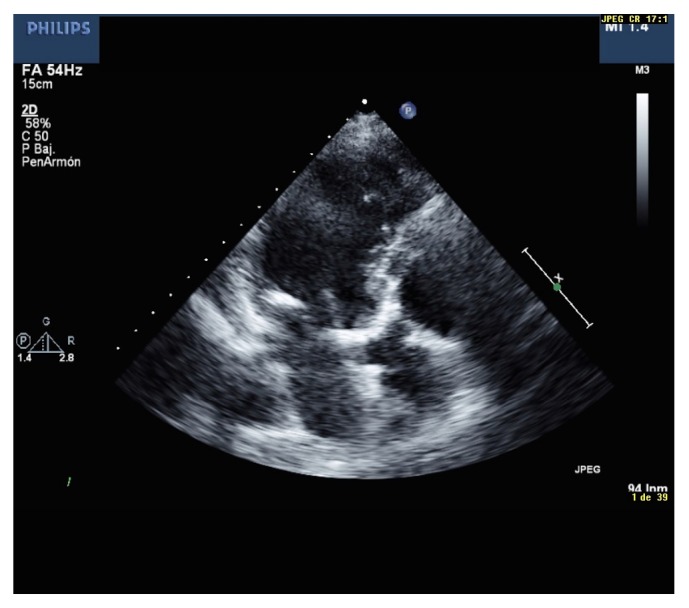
Transthoracic echocardiography, three-chamber view: intense calcification of aortic and mitral valves.
